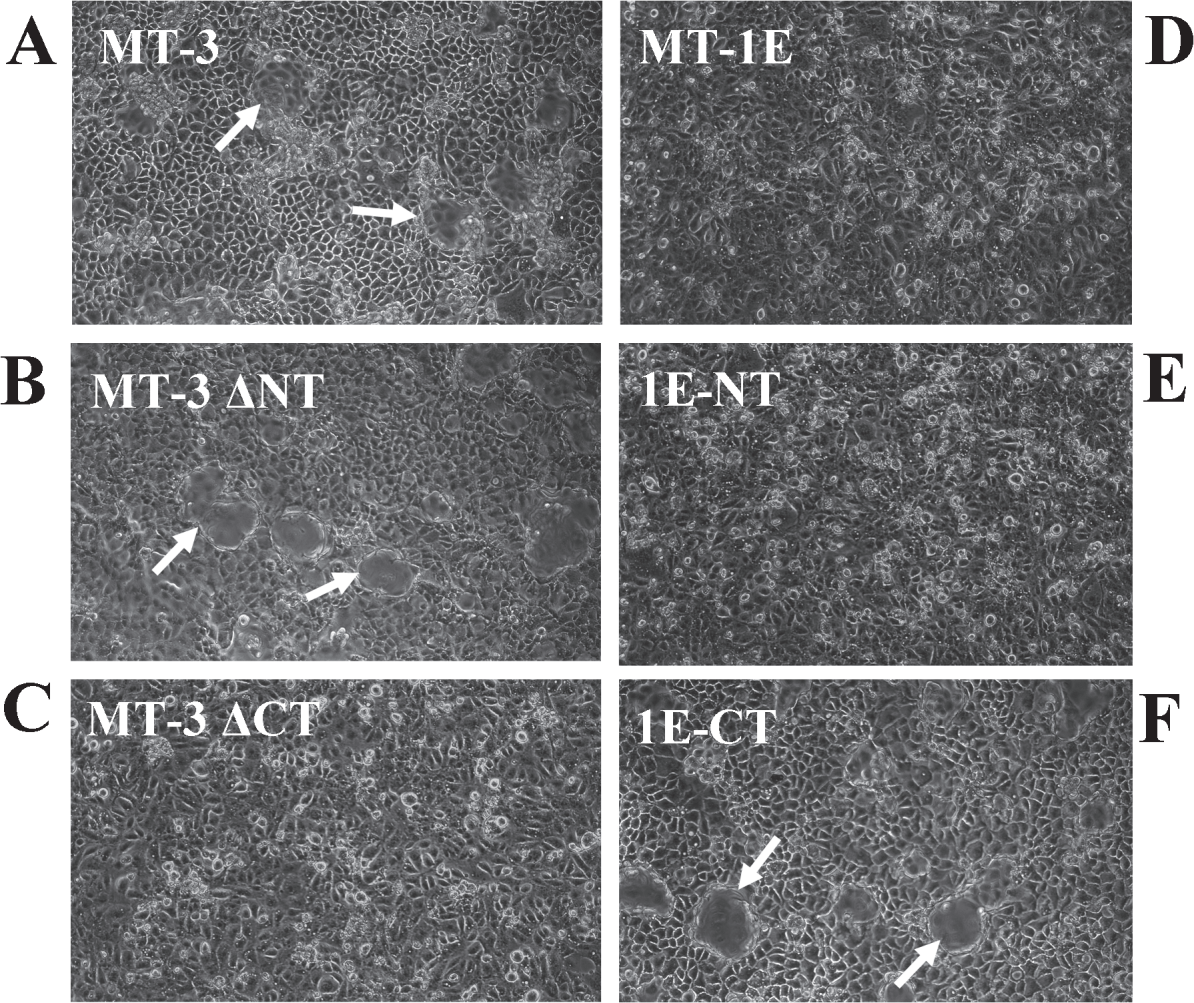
Effect of altered domains of metallothionein-3 on the formation of domes in HK-2 cells. To assess the domain of MT-3 that is responsible for the ability to confer dome formation, site-directed mutants of MT-3 were produced and the two unique domains of MT-3 were inserted into the non-doming MT isoform, MT-1E, as shown in Fig. 1. Each expression construct was stably transfected into HK-2 cells, and expressing clones were assessed for the ability to form domes. A) wild-type MT-3 showing dome formation when stably transfected, B) MT-3-ΔNT where the prolines in the N-terminal domain that confers growth inhibitory activity were converted to threonines, show that the ability to form domes was not compromised, C) MT-3ΔCT where the C-terminal EAAEAE sequence unique to the third isoform of metallothionein was deleted, shows the lack of dome formation, D) MT-1E, wild-type human metallothionein 1E, commonly expressed at high levels in many cell types exhibits no domes when stably transfected, E) 1E-NT, the N-terminal unique sequence of MT-3 was inserted into the corresponding position of MT-3 shows no conference of dome formation, and F) 1E-CT, the EAAEAE sequence of MT-3 was inserted in the corresponding position of MT-1E and when stably transfected into HK-2 cells confers dome formation.
